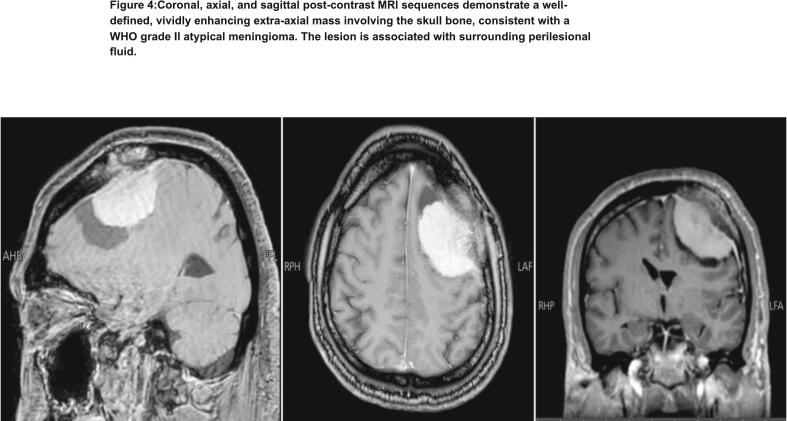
Coronal, axial, and sagittal post-contrast MRI sequences demonstrate a welldefined, vividly enhancing extra-axial mass involving the skull bone, consistent with a WHO grade II atypical meningioma. The lesion is associated with surrounding perilesional fluid.
